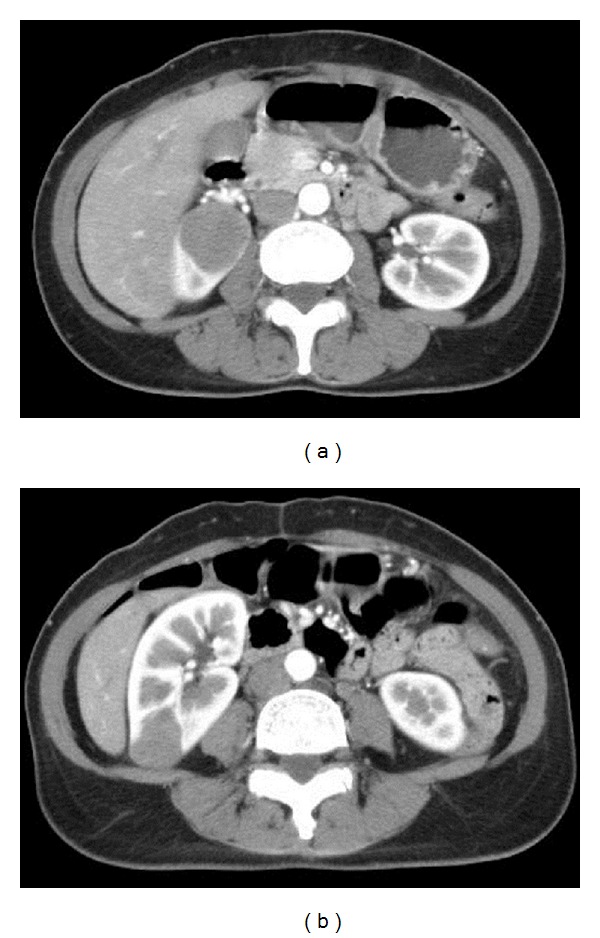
Abdominal computed tomography (CT) scan. Abdominal CT scan enhancement showed hypovascular tumors at upper poles (a), lower poles (b), and middle portions of the right kidney.
